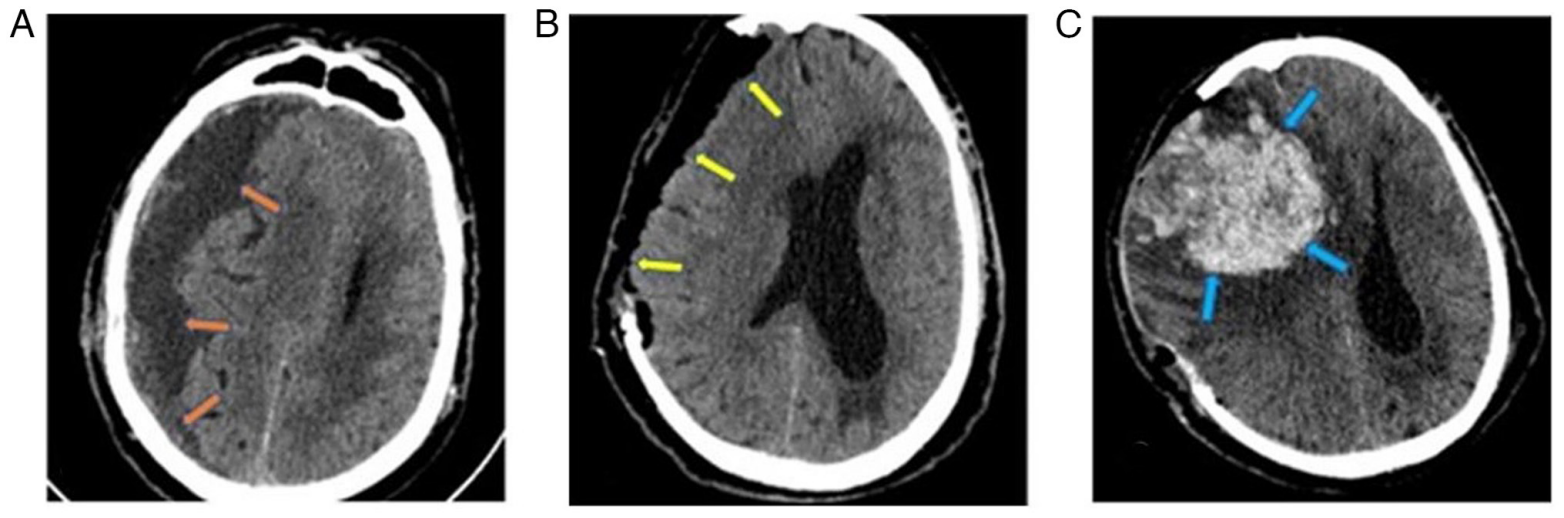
(A) A head CT scan at 2 months after the initial diagnosis revealed a second hematoma recurrence (orange arrows) with a significant mass effect. (B) The patient underwent craniectomy, membranectomy and hematoma evacuation (yellow arrows). (C) The CT scan on the 2nd post-operative day revealed a massive intraparenchymal hemorrhage (blue arrows) and significant edema. CT, computed tomography.
